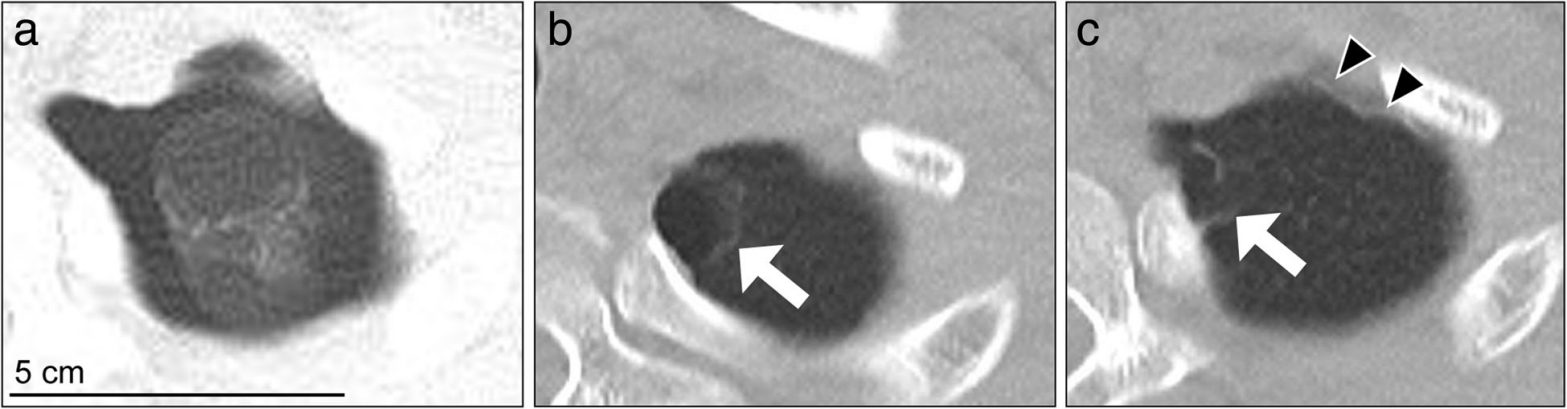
Chest computed tomography scan showing typical neogenetic bullae neighboring the staple line. a Preoperative findings of the pulmonary apex: the left side preoperative pneumothorax can be seen, but the bullae on the apex that are detected postoperatively are not visible. b One-year postoperative findings clearly show bullae on the apex. c At a higher magnification, the staple line can be seen. The white arrow indicates the neogenetic bullae, while the black arrowheads indicate the staple lines
